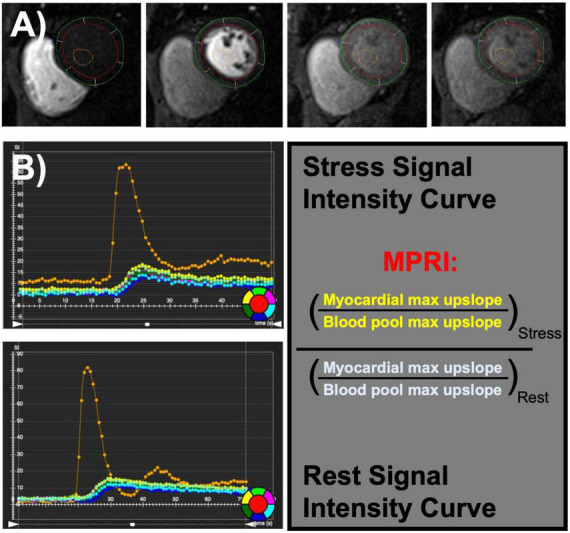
Coronary microvascular dysfunction can be assessed using semi-quantitative evaluation of first-pass perfusion images (A) during stress and rest cardiac magnetic resonance imaging (MRI). Myocardial perfusion reserve index (MPRI) is calculated by the maximal upslope of myocardial intensity normalized by maximal upslope of blood pool intensity at stress divided by rest (B).
